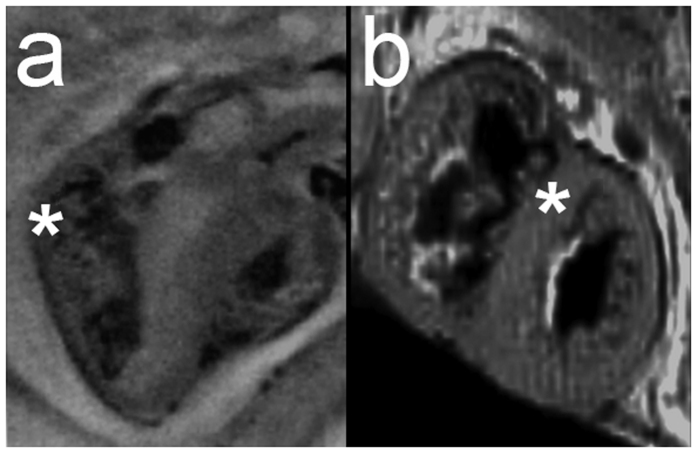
Crypts in developing human hearts. (a) A basal inferolateral crypt can be seen in this 3-chamber view of a CS21 human heart by OPT (asterix); (b) a mid anteroseptal crypt is seen in this short-axis view of a 9 pcw human fetus by MRI (asterix). CS = Carnegie stage; MRI = magnetic resonance imaging; OPT = optical projection tomography; pcw = post-coital week.
